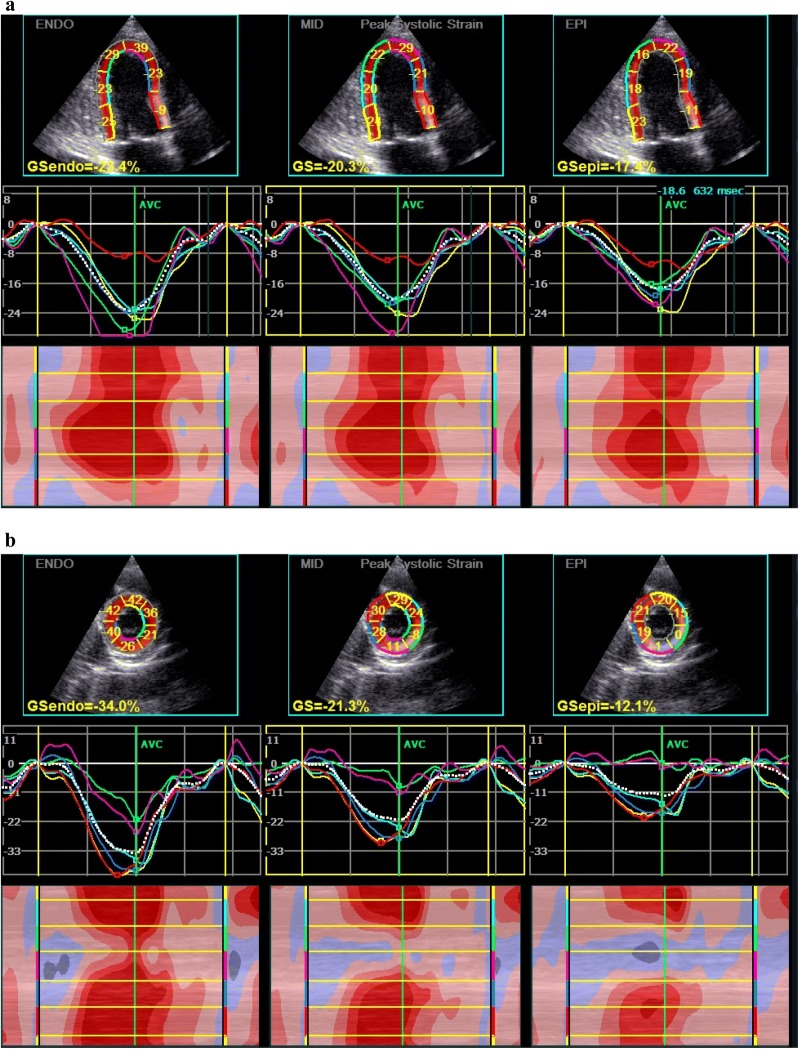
Multilayer longitudinal and circumferential strains determined by two-dimensional speckle tracking echocardiography (2D-STE) in diabetic patient with acute hyperglycemia. a Subendocardial (Endo), mid-myocardial(Mid) and subepicardial(Epi) layers of longitudinal strain assessed in apical long-axis views; b Endo, Mid and Epi layers of circumferential strain at base (mitral valve) evaluated in parasternal short-axis view
